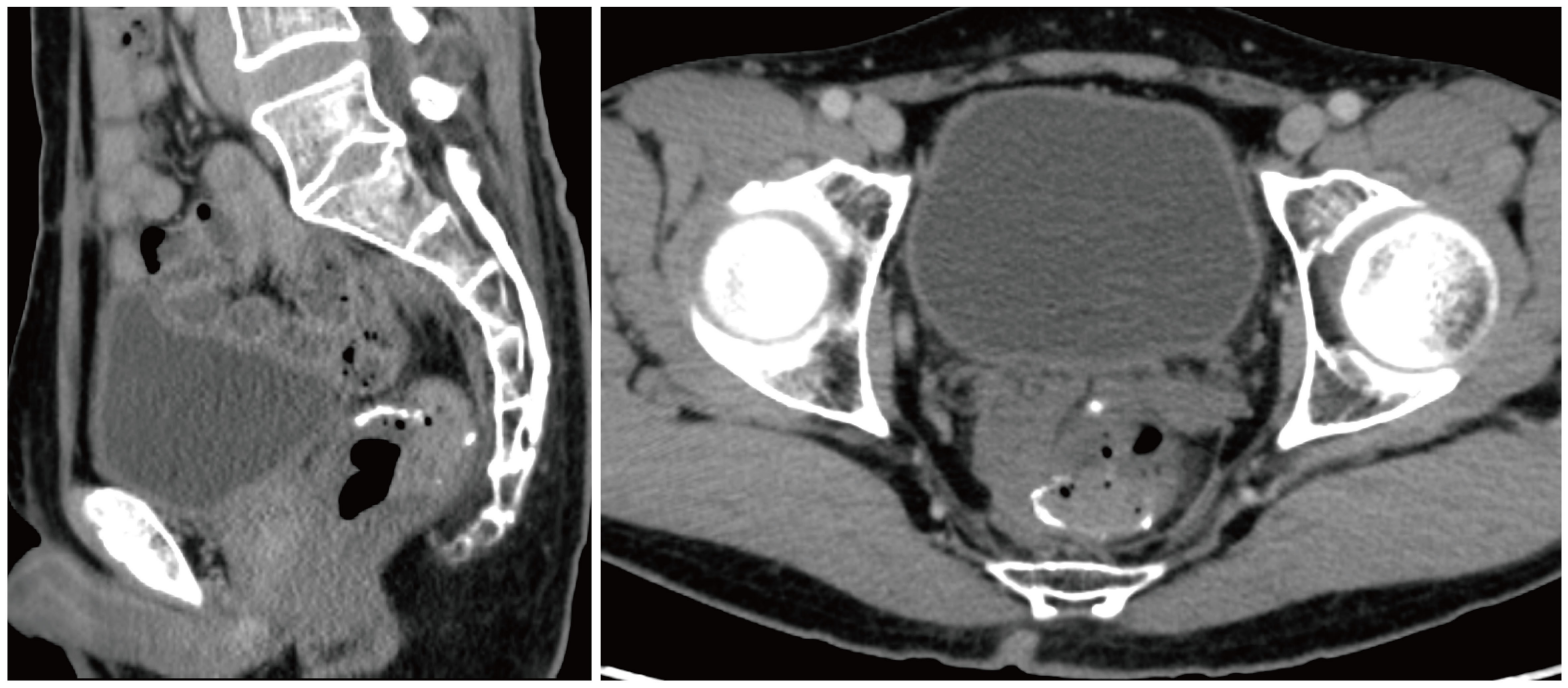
Enhanced CT examination suggested no ascites or recurrence.
